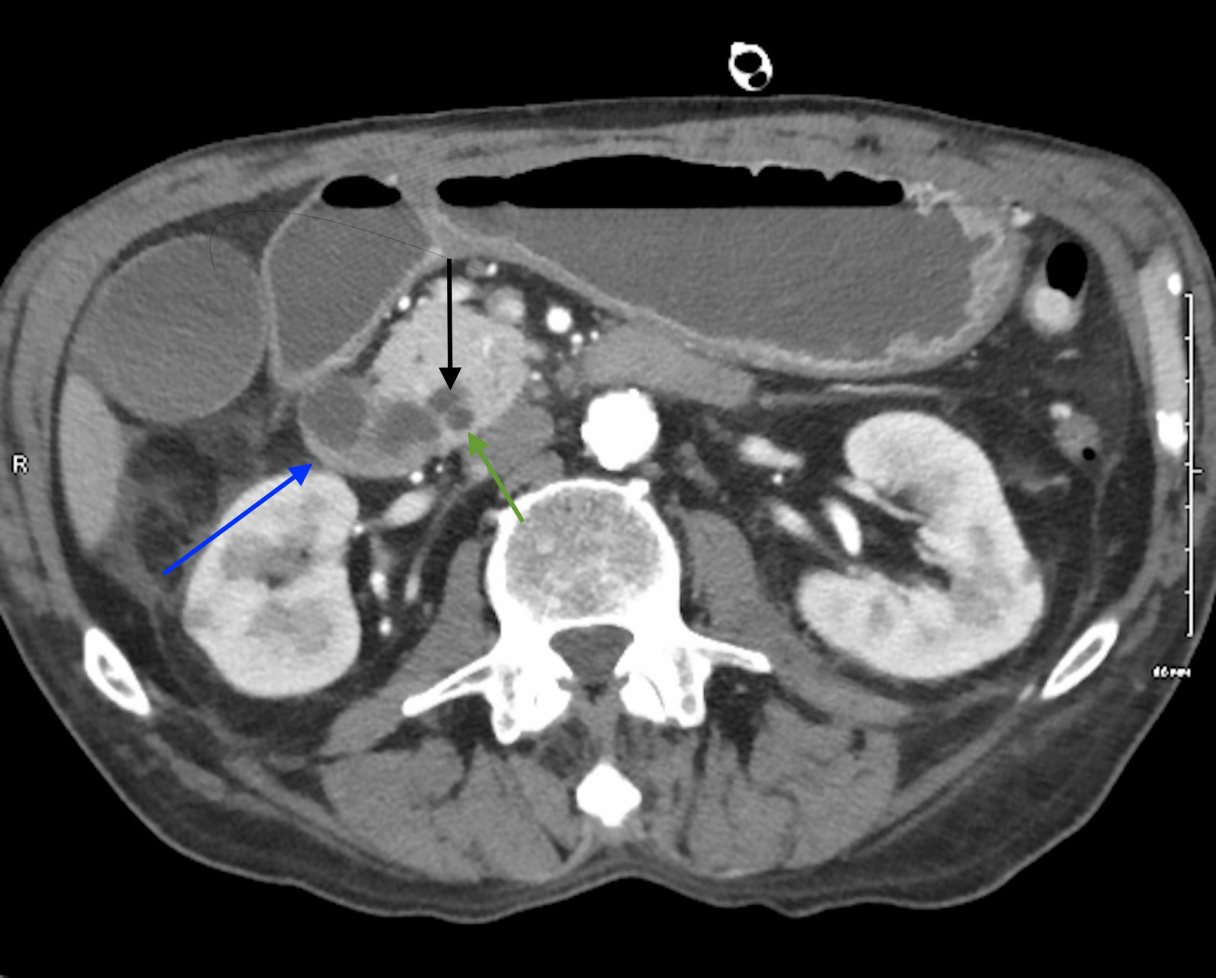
Axial view abdominal CT scan in the arterial phase Blue arrow: showing a multicystic mass; Black arrow: showing common bile duct measuring 5 mm; Green arrow: showing main pancreatic duct measuring 6 mm.
